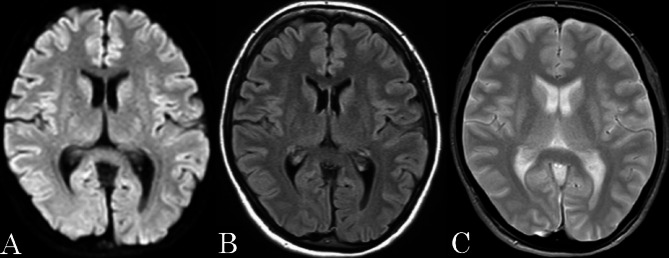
Brain magnetic resonance imaging on the day 3. A: Diffusion-weighted image. B: Fluid-attenuated Inversion recovery. C: T2 star-weighted image. There were no abnormalities in the brain parenchyma, or thrombosed vein sign
